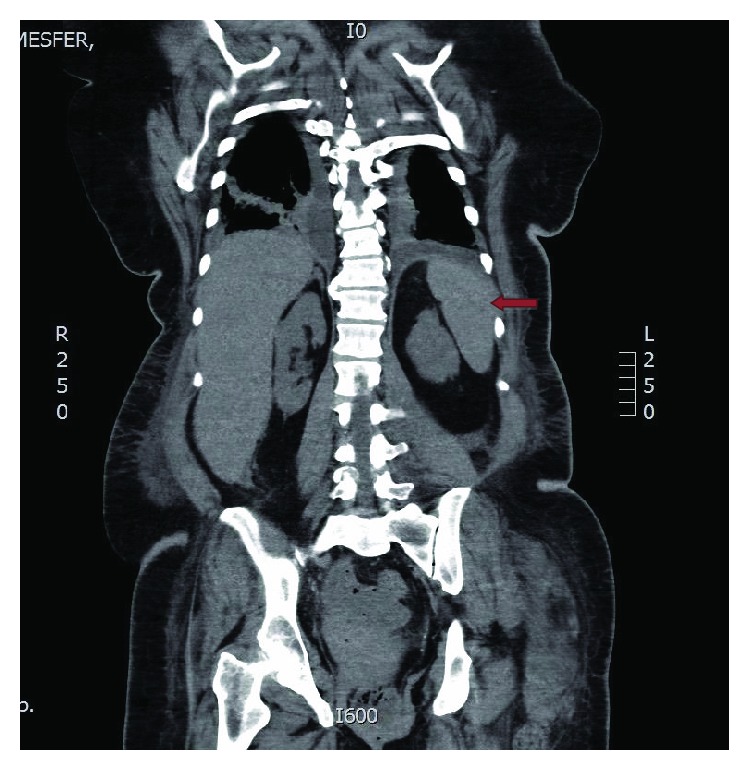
CT scan of the abdomen with IV contrast showing a normal spleen (red arrow).
